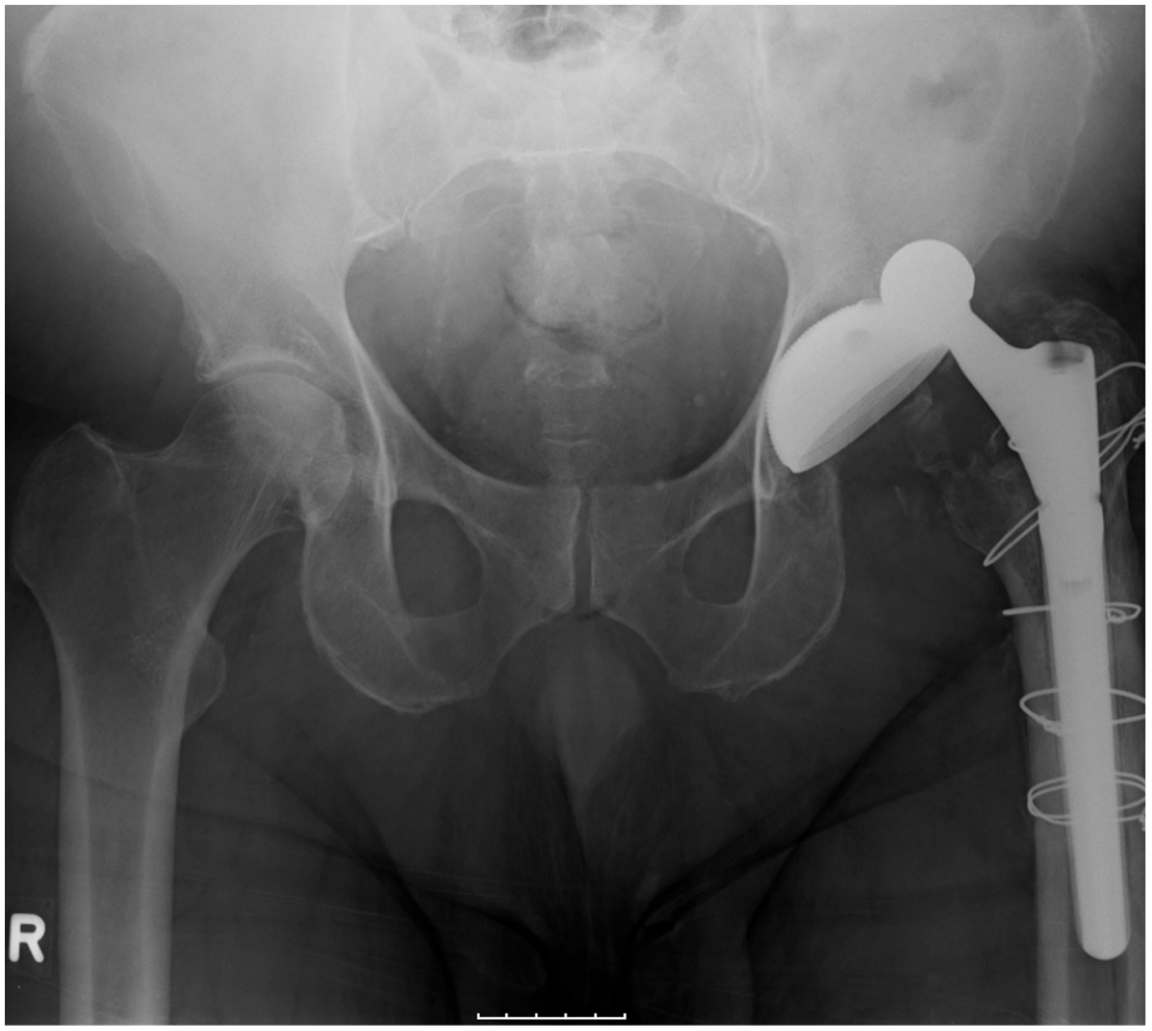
58 year old man presenting with hip pain. Conventional x-ray showed a dislocated total hip replacement on the left.
